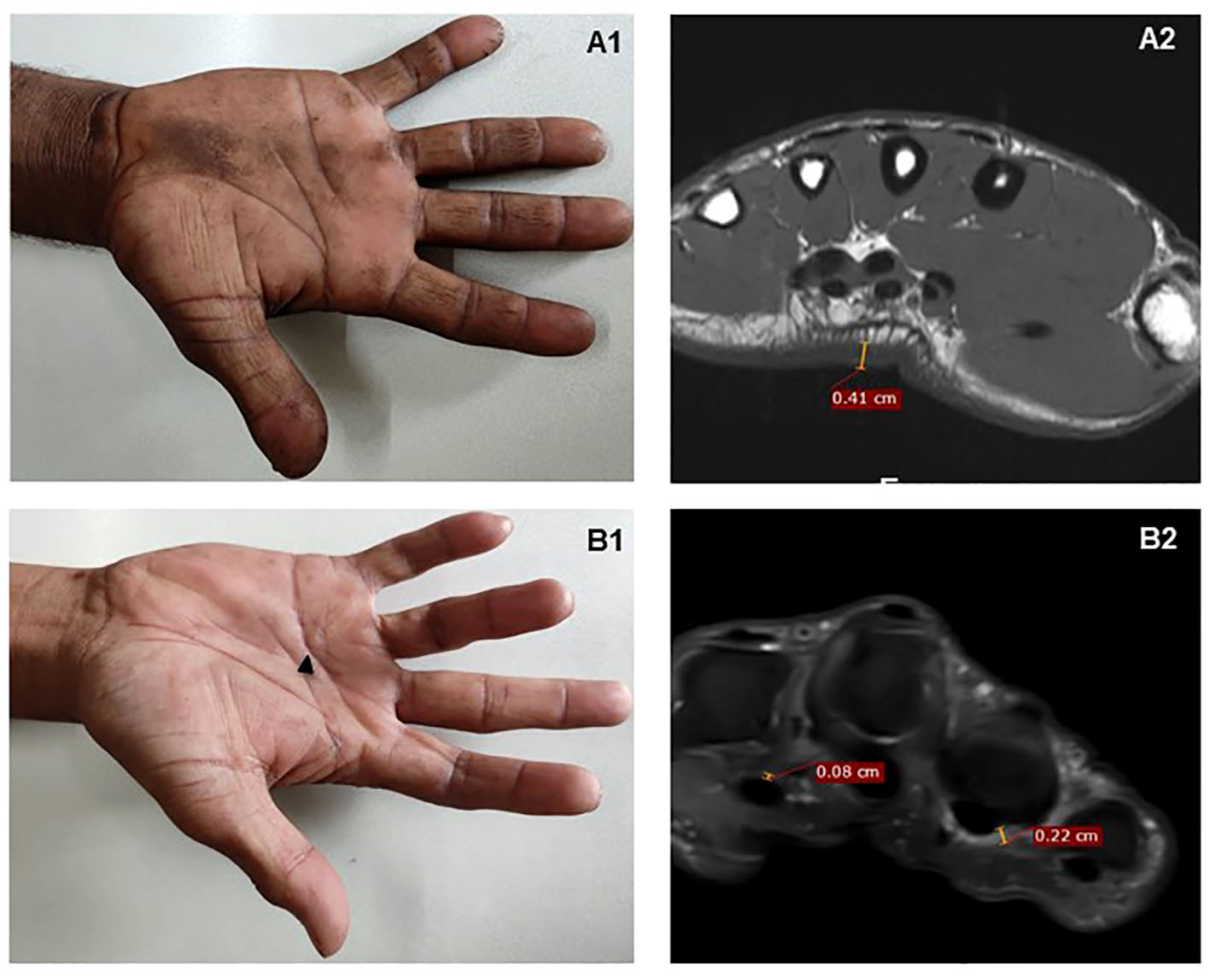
MRI findings in two patients with MCP extension restriction. (A1) A patient with severely restricted MCP extension – mean 10°. (A2) MRI demonstrates considerable palmar skin thickening alone on T1-weighted axial images, seen at mid-metacarpal level; there is no tenosynovial or palmar fascia thickening. (B1) A patient with bilateral Dupuytren’s contracture on the fourth finger (black arrow); (B2) PDFS scan shows ill-defined PDFS hyperintense soft tissue thickening in relation to flexor thickening also involving A1 pulley and the palmar fascia.
